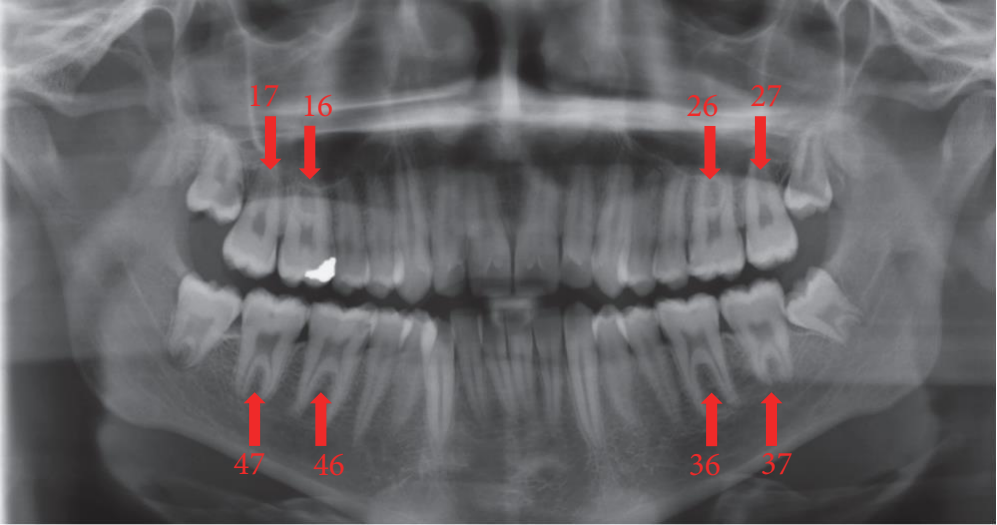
Orthopantomogram showing bilateral taurodontism affecting maxillary second and first molars (17, 16, 26, 27) and mandibular first and second molars (37, 36, 46, 47).
